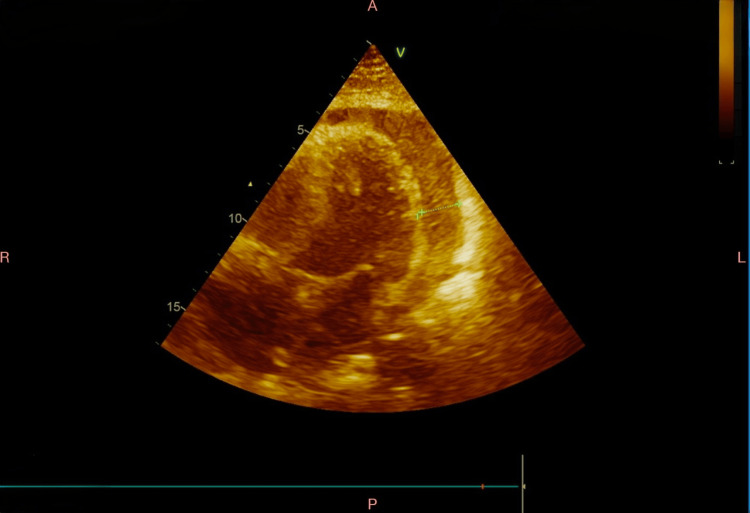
Two-dimensional echocardiogram demonstrating moderate pericardial effusion.
